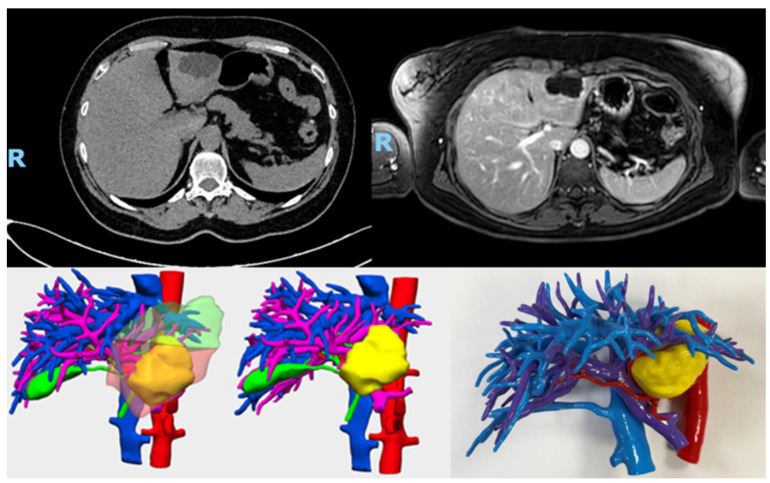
Virtual and printed 3D model overlaid on the 2D imaging (CT and MRI) of a cystic lesion in segments 2–3.
